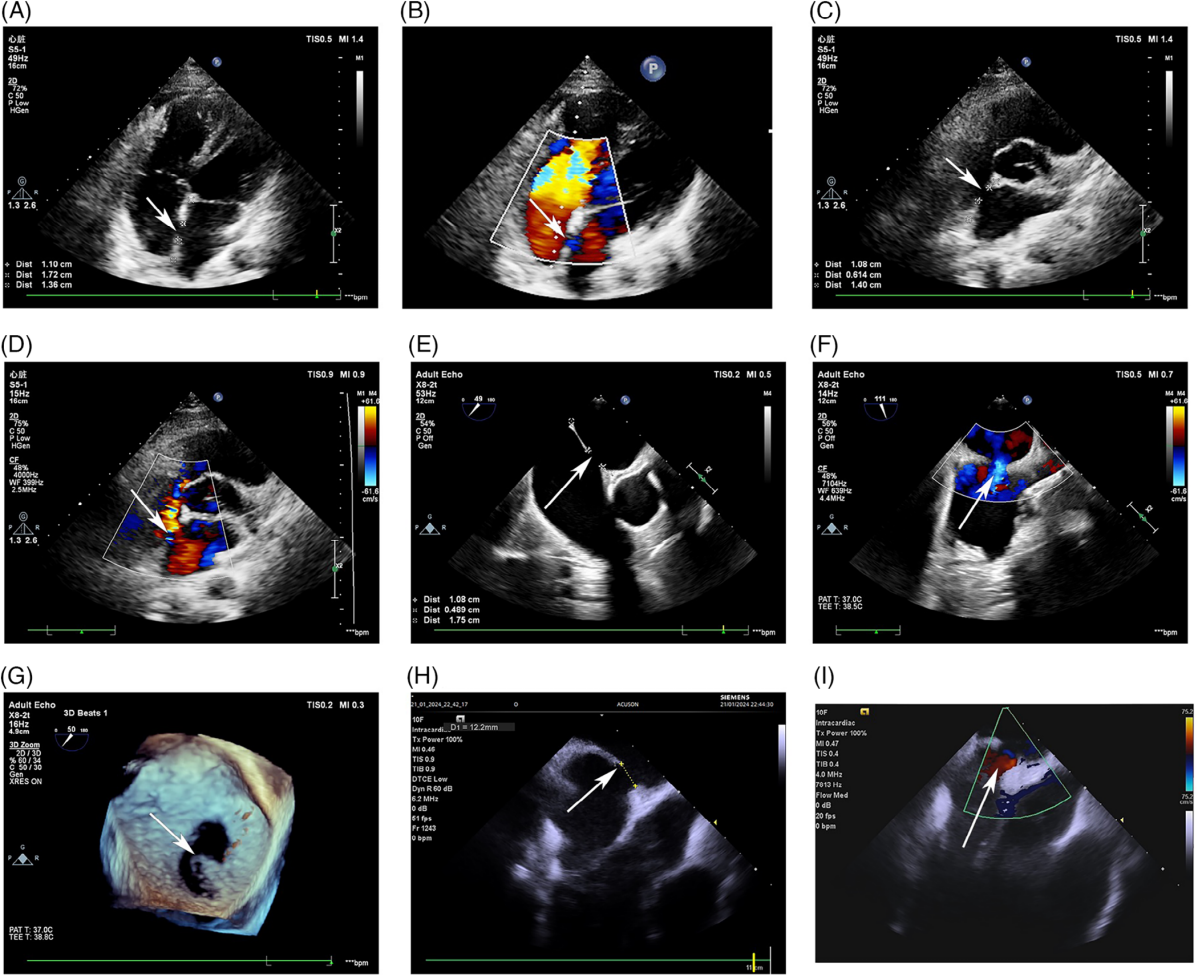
Echocardiographic images of atrial septal defects (ASDs). (A) Two‐dimensional transthoracic echocardiography (TTE) imaging of ASD in four‐chamber view. (B) Color Doppler flow imaging of ASD in four‐chamber view. (C) Two‐dimensional TTE imaging of ASD in subcostal view. (D) Color Doppler flow imaging of ASD in subcostal view. (E) Two‐dimensional transesophageal echocardiography (TEE) imaging of ASD. (F) Transesophageal color Doppler flow imaging of ASD. (G) Transesophageal three‐dimensional echocardiographic imaging of ASD. (H) Intracardiac echocardiography (ICE) imaging of ASD. (I) Intracardiac color Doppler flow imaging of ASD. Arrows point to the ASD.
